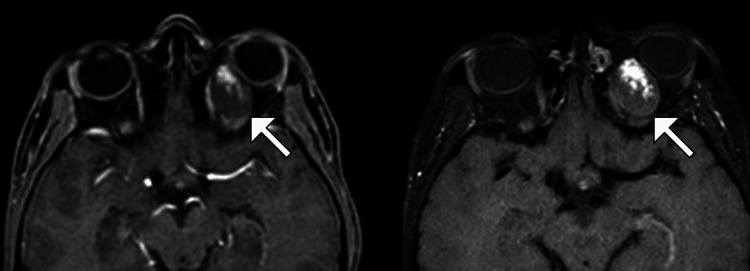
Axial T1-weighted MRI with contrast showing a well-defined left intraconal lesion with heterogeneous progressive enhancement (arrows) The lesion is displacing adjacent orbital structures, including the optic nerve, but without evidence of direct invasion, consistent with the imaging characteristics of a cavernous hemangioma.
